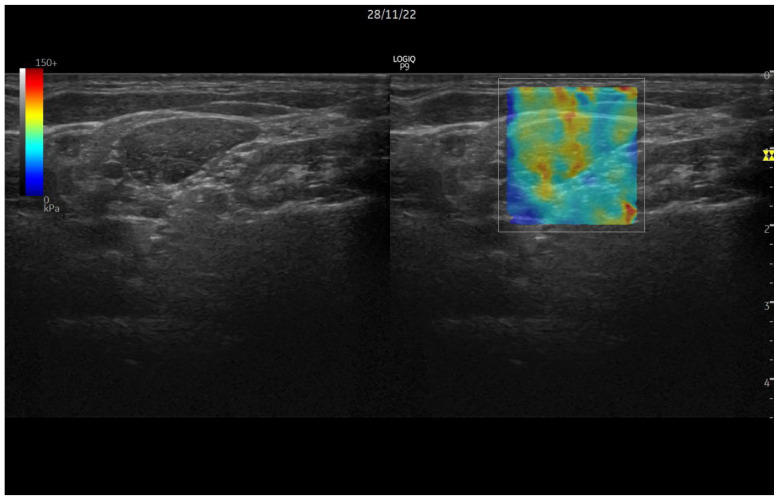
Sonoelastography ultrasound.
